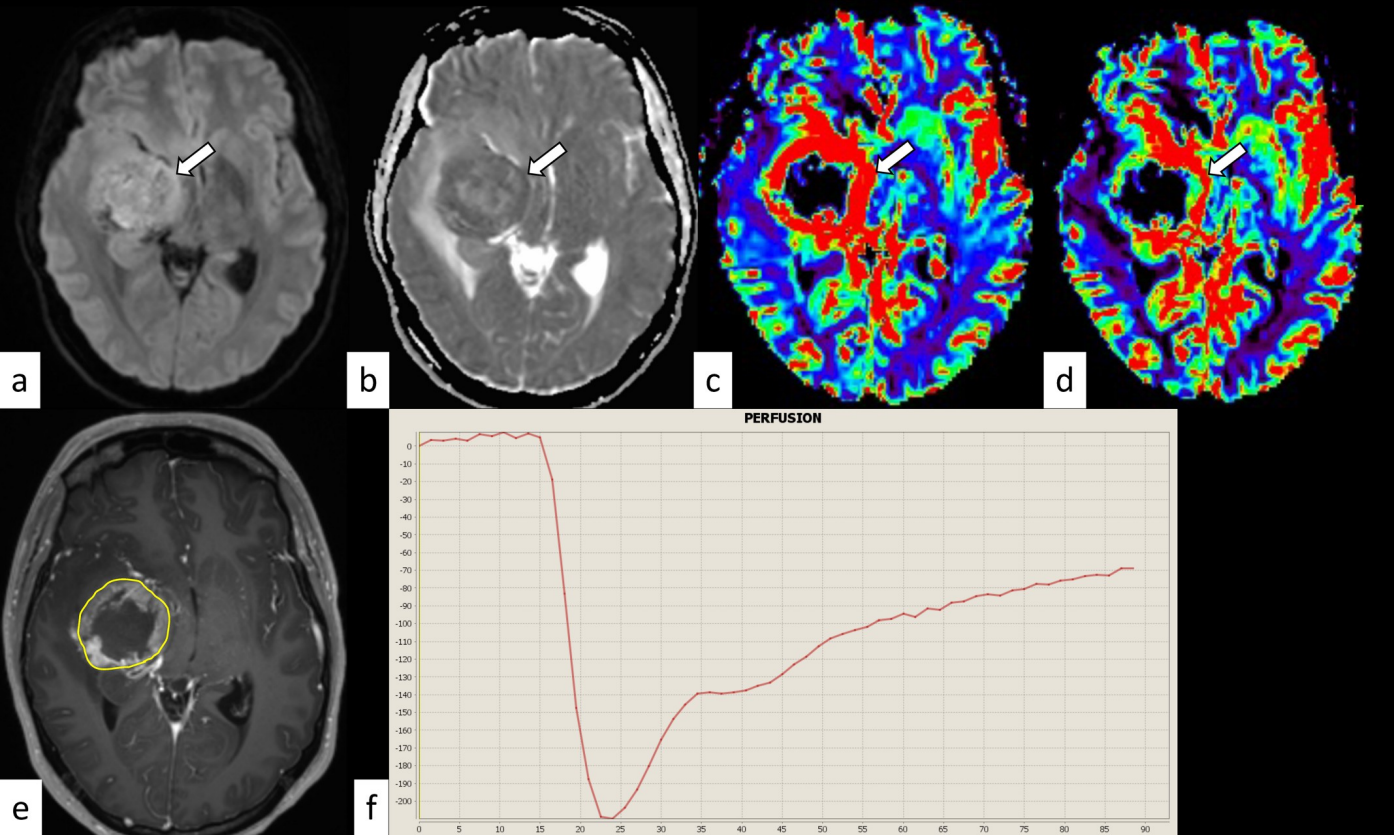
H3.3 variant diffuse midline glioma, H3K27-altered in a 28-year-old woman. The mass shows hyperintensity on diffusion-weighted imaging (a, arrow) and a decreased apparent diffusion coefficient (nADCmean is 1.18) (b, arrow). The normalized relative cerebral blood volume and flow are 2.93 and 2.10, respectively (c and d, arrows). The corresponding post-contrast T1-weighted image and region-of-interest in this slice are shown (e). The percentage signal recovery of the mass is 0.63 (f). nADCmean = normalized mean apparent diffusion coefficient.
